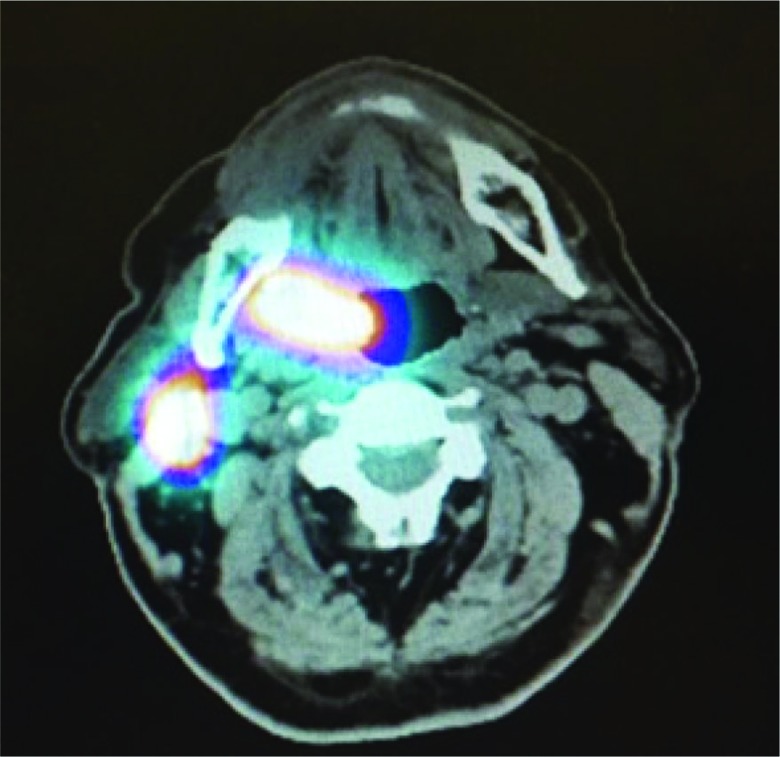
SPECT/CT and lymphoscintigraphy fusion. Right tonsillar tumor with a sentinel lymph node at level II on the right side.
